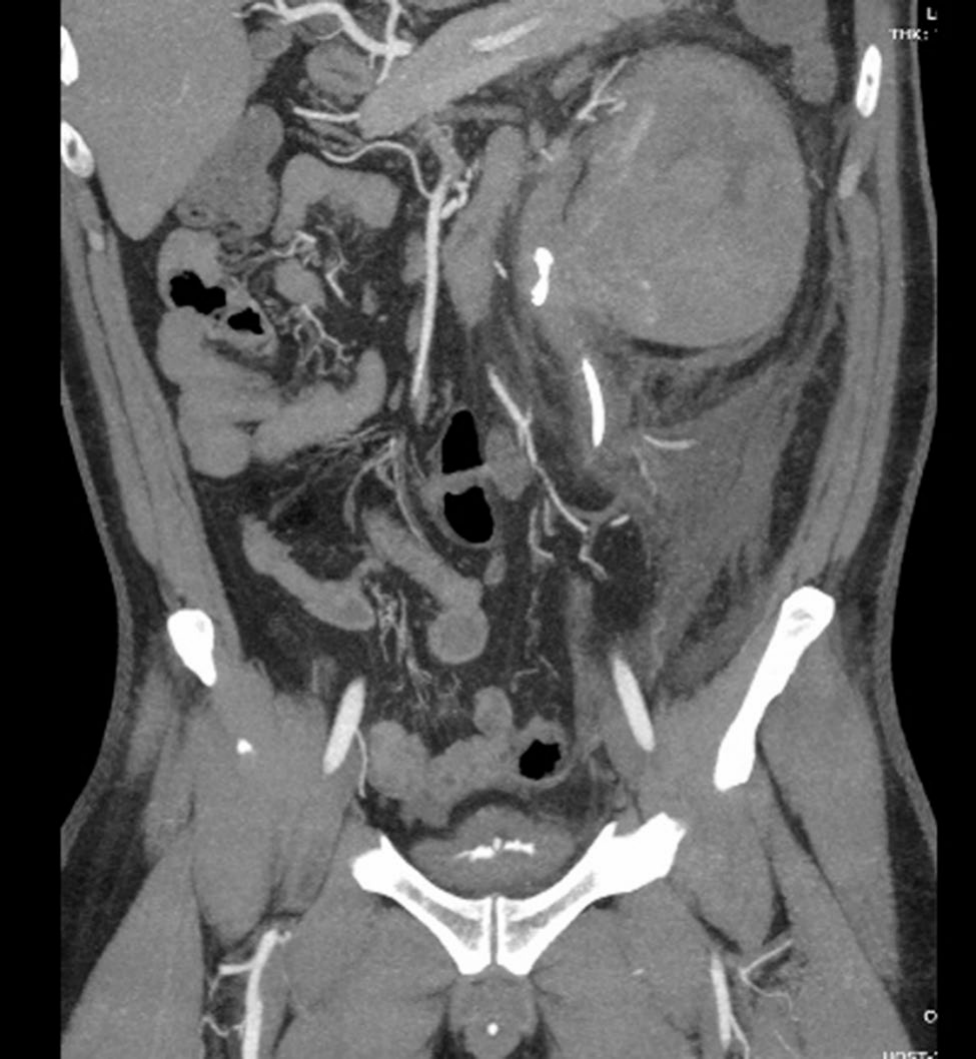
Postoperative computed tomography demonstrating a massive perinephric hematoma
